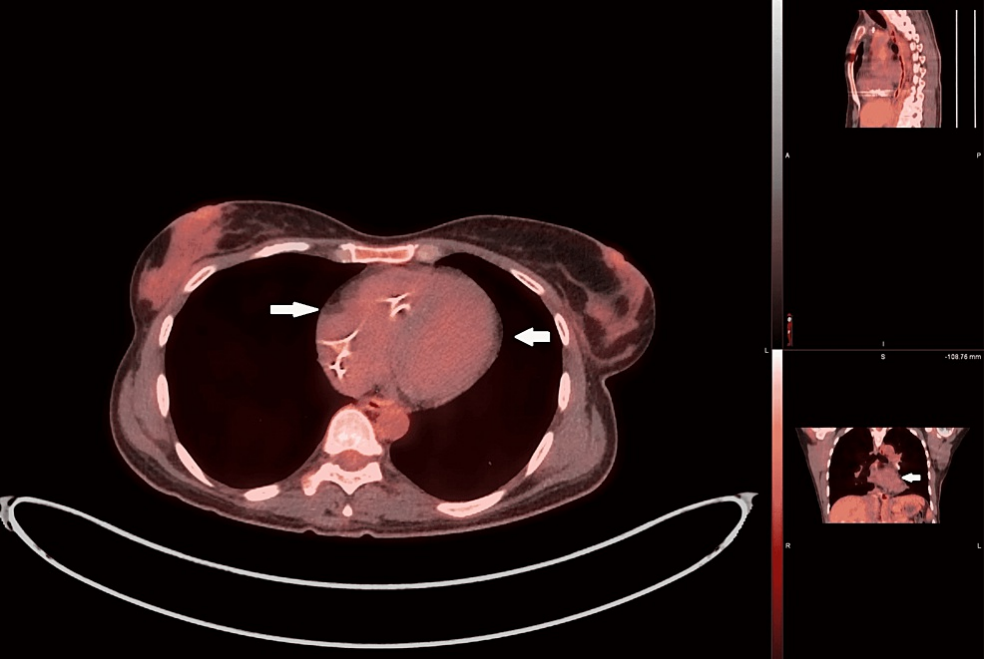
Cardiac PET scan six months post-treatment initiation reveals resolution of FDG-PET uptake as highlighted by white arrows FDG: fluorodeoxyglucose; PET: positron emission tomography.
